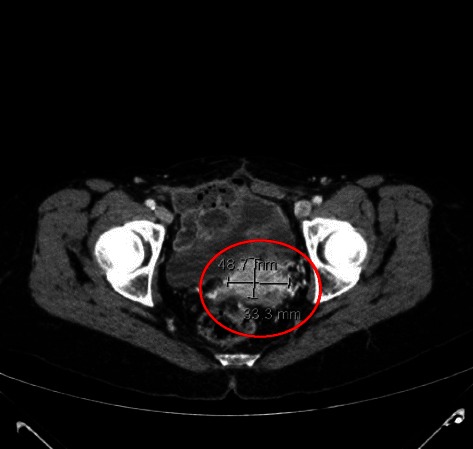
September 2013: CT scan showing abdominal progression with an increase in the size of the peritoneal lesions
